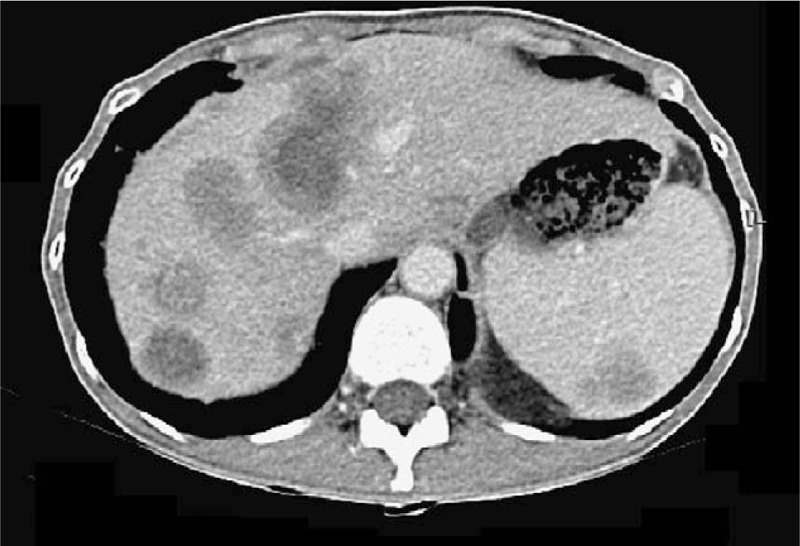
Follow-up CT scan 6 months after the diagnosis of breast metastasis showed evidence of progressive disease (RECIST 1.1 criteria^[47]^). The largest hepatic lesion was found 4.0 cm in size.
